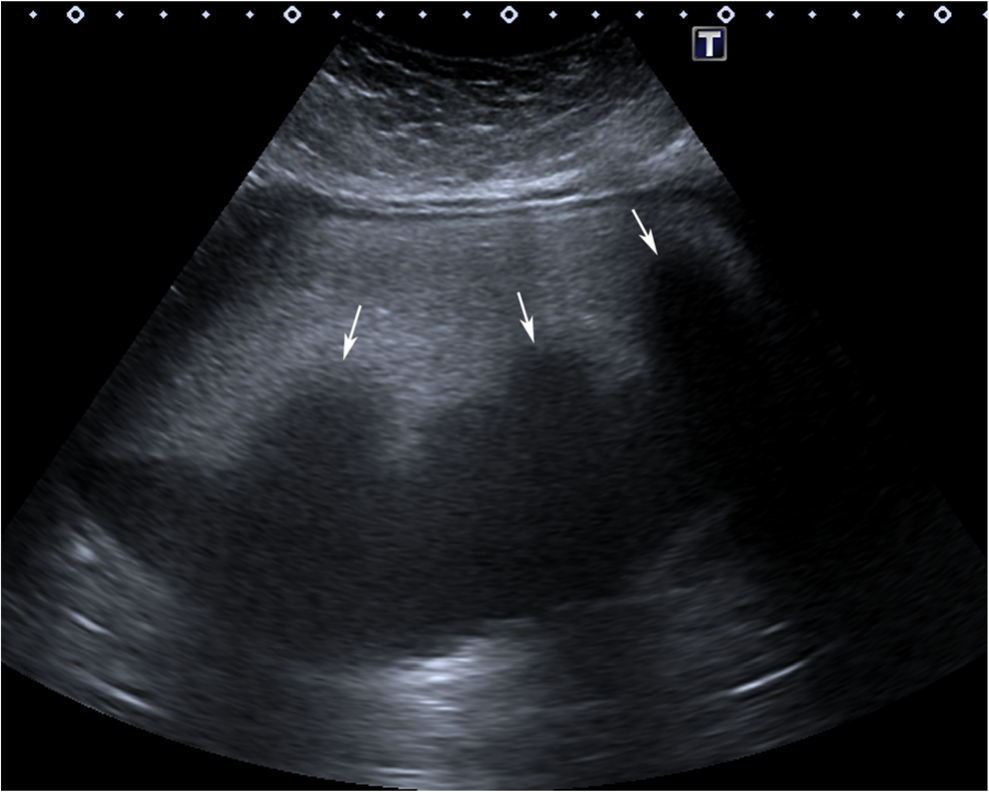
Transverse sonogram of an 18-year-old female with a mature cystic teratoma. There are three dark comet tail appearances (arrows) floating in the interface of the fat-fluid level consistent with hair balls pathologically
